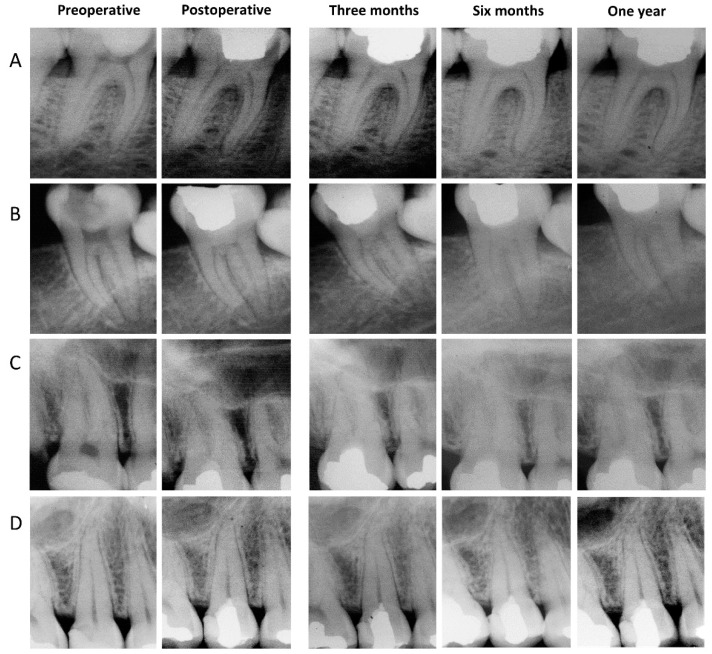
Preoperative, postoperative, and the three follow-up (3, 6, and 12 months) radiographs of four representative cases. None of them presented pain, tenderness to percussion or palpation, tooth discoloration, abnormal mobility, swelling, periodontal pocket, sinus tract, nor evidence of periapical radiolucency, furcal pathosis, or root resorption.
